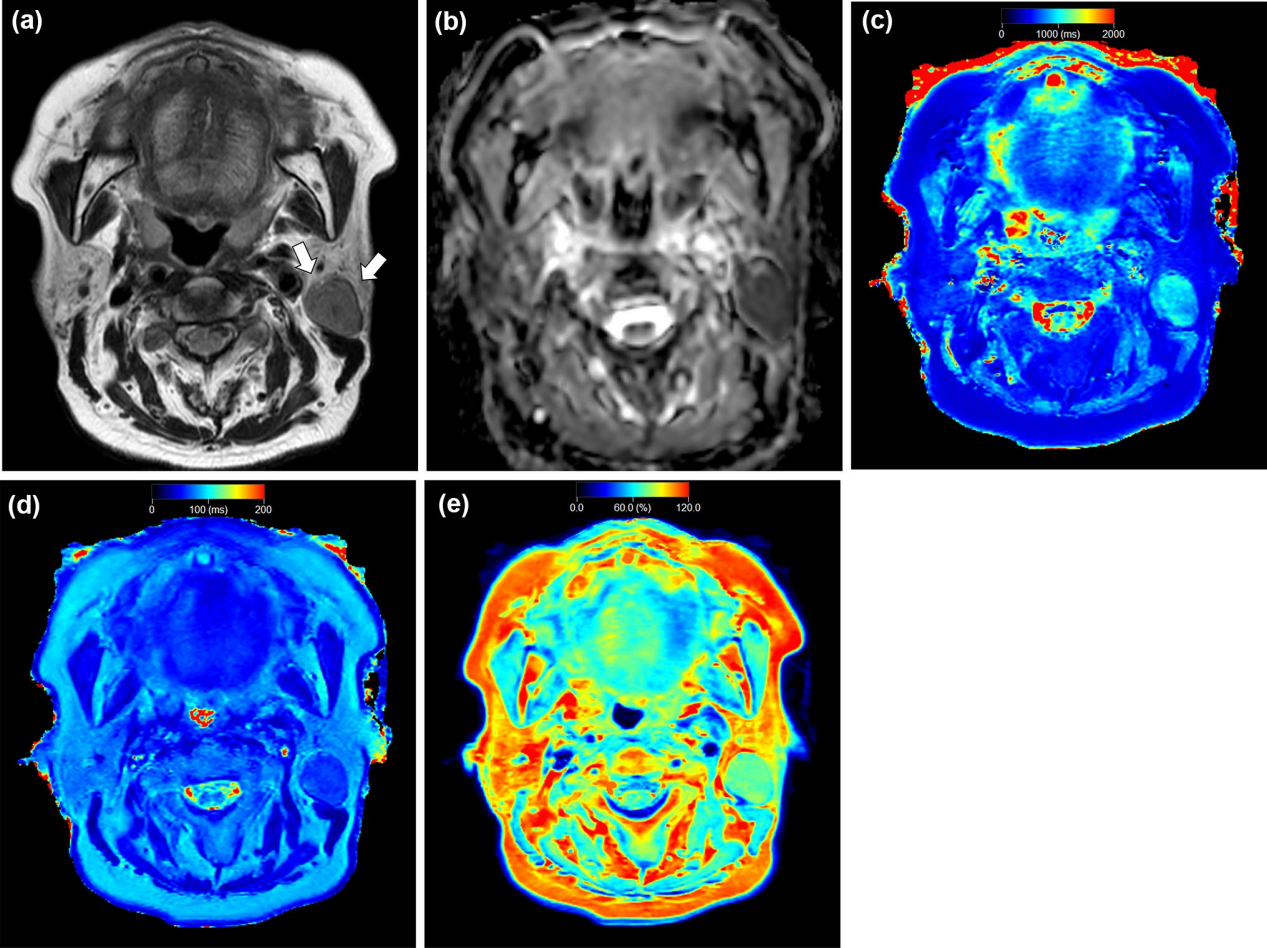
A 65-year-old female with Warthin tumor. T2-weighted image (a) shows a lesion with lower signal intensity (arrows) relative to parenchyma in the adjacent left parotid gland. Apparent diffusion coefficient (ADC) (b), T1 (c), T2 (d), and PD (e) maps show the lesion, which has a mean ADC value of 0.83 × 10^−3^ mm^2^/s, mean T1 value of 926 ms, mean T2 value of 62 ms, and mean PD value of 73%
